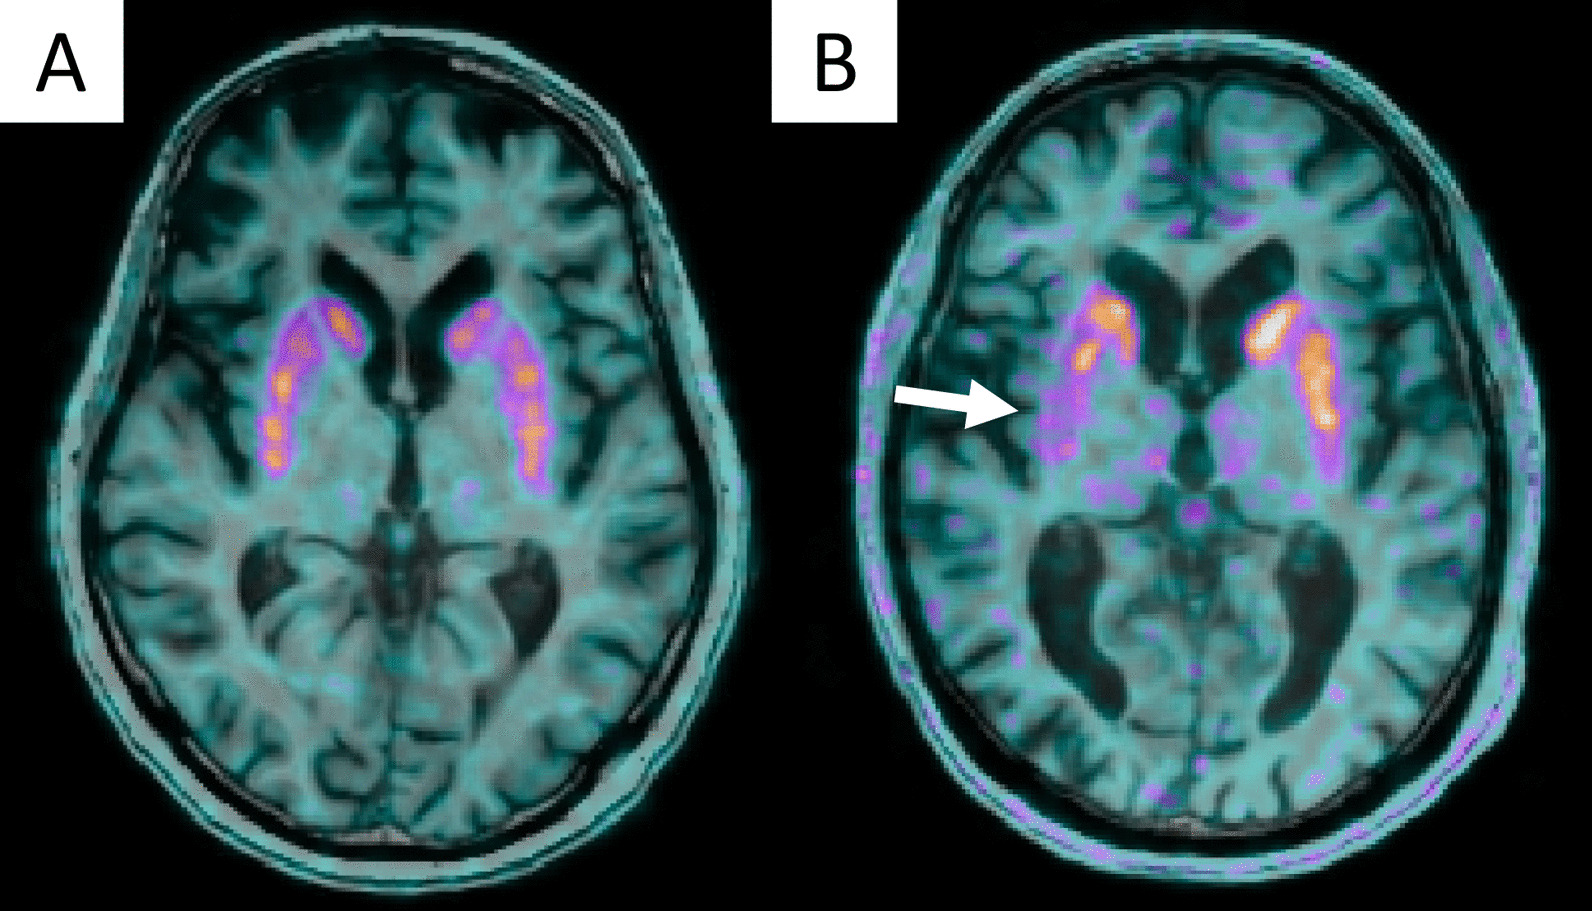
Visual analyses of ^18^F-DOPA PET/MRI according to the international guidelines [11]. A This IPD patient, visually considered normal. The clinical follow-up and had symptoms relief after L-DOPA therapeutic test classified him as FN. Only min SUVr was below the 95% confidence interval estimated from controls. B This IPD presented unilateral right posterior putamen (white arrow) pre-synaptic dopamine denervation and global striatal ^18^F-DOPA decrease with faintly increased background unspecific activity. PET right putamen dopamine denervation was confirmed with symptoms relief after L-DOPA therapeutic test
